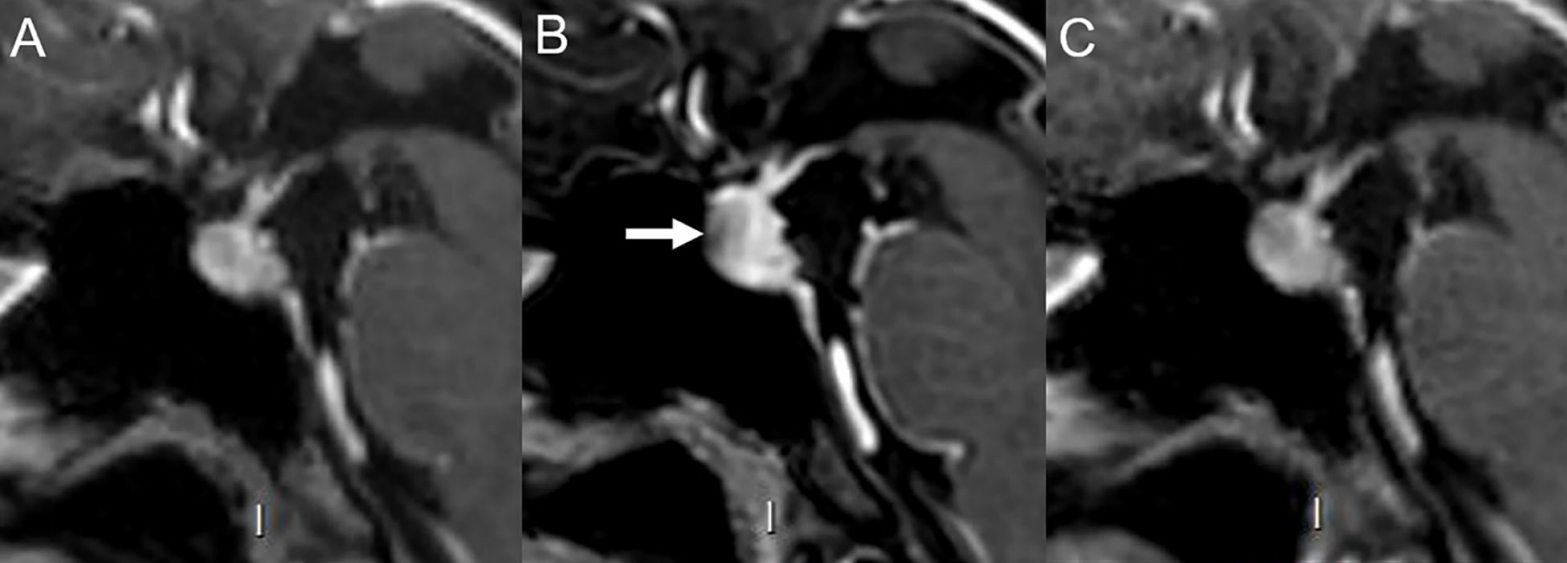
Immune-related hypophysitis in a 43-year-old female with cutaneous melanoma treated with ipilimumab and nivolumab. (A) Normal hypophyseal MRI performed 4 months prior to immunotherapy. After 3 cycles of ipilimumab (anti-CTLA-4) and nivolumab (anti-PD-1), the patient developed headaches. (B) MRI revealed increased hypophyseal height with mild pituitary stalk thickening and reduced opto-chiasmatic cistern size compatible with hypophysitis (arrow). Ipilimumab was discontinued and 2 more cycles of nivolumab alone were administered. Eventually, nivolumab was discontinued due to a grade 3 toxidermia. (C) Hypophyseal MRI performed 3 months after the last immunotherapy cycle was normal with disappearance of signs of inflammation.
